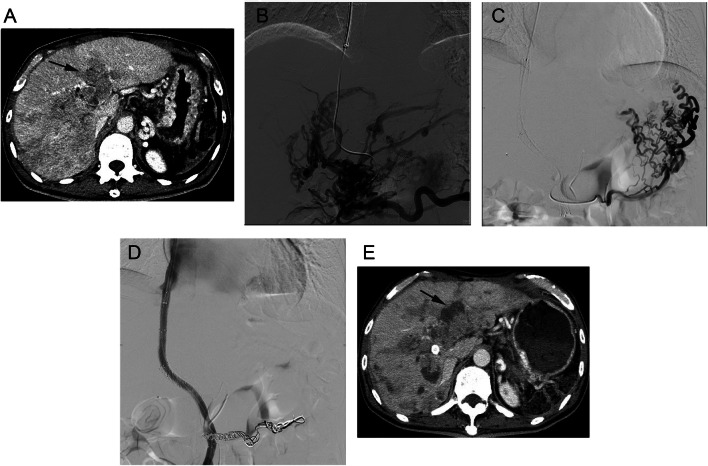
A 55-year-old male patient with hepatocellular carcinoma (HCC) and tumour thrombus-related symptomatic portal hypertension underwent a transjugular intrahepatic portosystemic shunt (TIPS) procedure for severe variceal bleeding and received sequential systemic therapy with lenvatinib (8 mg/qd). A Contrast-enhanced computed tomography showed advanced HCC with extensive portal vein tumour thrombus (PVTT) before treatment (black arrowhead). B Superior mesenteric venography showed the disappearance of the portal vein and branches that were replaced by disordered collateral veins. The portal venous pressure gradient (PPG) was 28.2 mmHg before TIPS creation. C Angiography showed gastroesophageal varices (GOV2). D After TIPS creation, angiography showed that the stent was smooth, and the collateral circulation veins were significantly reduced. The portal venous PPG was 11.5 mmHg after TIPS creation. The gastroesophageal varices were embolised with coils, and the varices disappeared on angiography. E After 6 months of sequential systemic therapy, the viable lesions of the tumour and PVTT were significantly decreased (black arrowhead), and the TIPS patency was satisfactory. The overall survival period after TIPS placement was 10.8 months without variceal rebleeding
